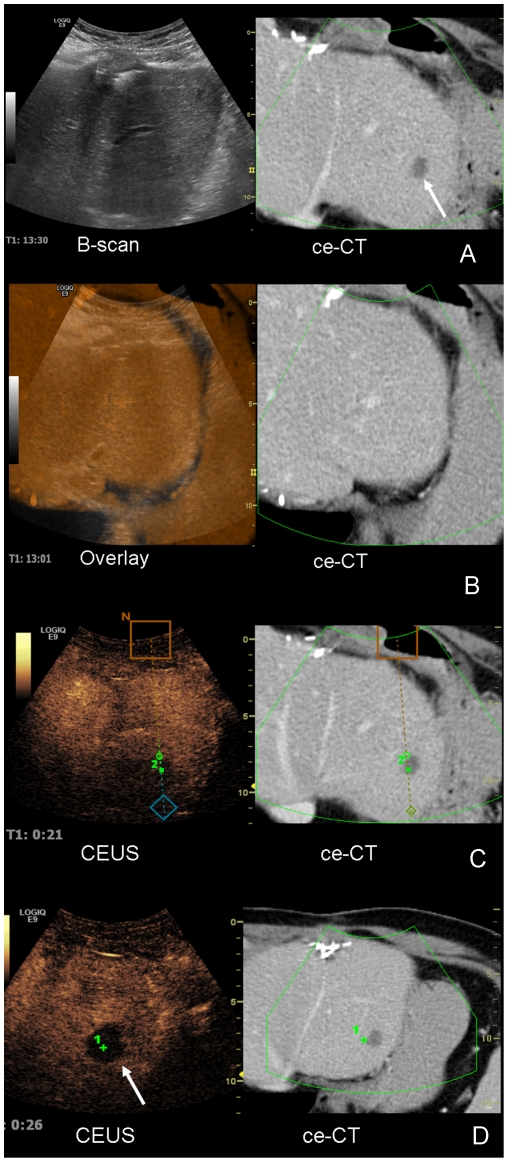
Image fusion (ultrasound and CT) for interventional planning for local radiofrequency ablation. A 67 years old patient with colorectal carcinoma and several partial liver resections in his history showed a new solitary liver metastasis in segment II of the liver, clearly visible in ceCT. The referring surgeons requested a local radiofrequency ablation of the metastasis. Figure 2 A. The metastasis cannot be detected in fundamental B-scan, but in the ceCT on the right side. Figure 2 B. For image fusion the contrast enhanced CT scan is color-coded and superimposed onto the fundamental B-scan. Figure 2 C. CEUS clearly shows the metastasis, and is therefore used for planning of the radiofrequency ablation. Figure 2 D. CEUS control after radiofrequency ablation with point registration shows complete necrosis in the area of the former metastasis with a safety margin of over 1 cm in all directions.
